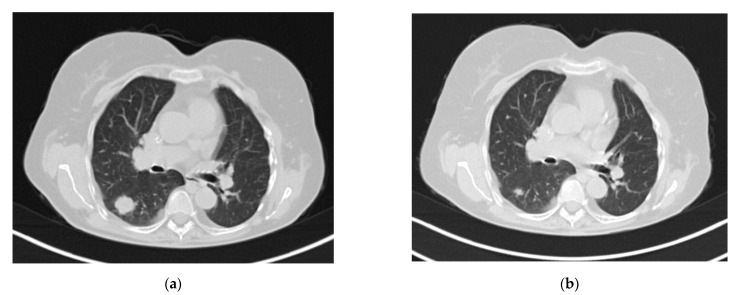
(a) Presentation CT scan of Case 2; (b) CT scan after antifungal treatment.
